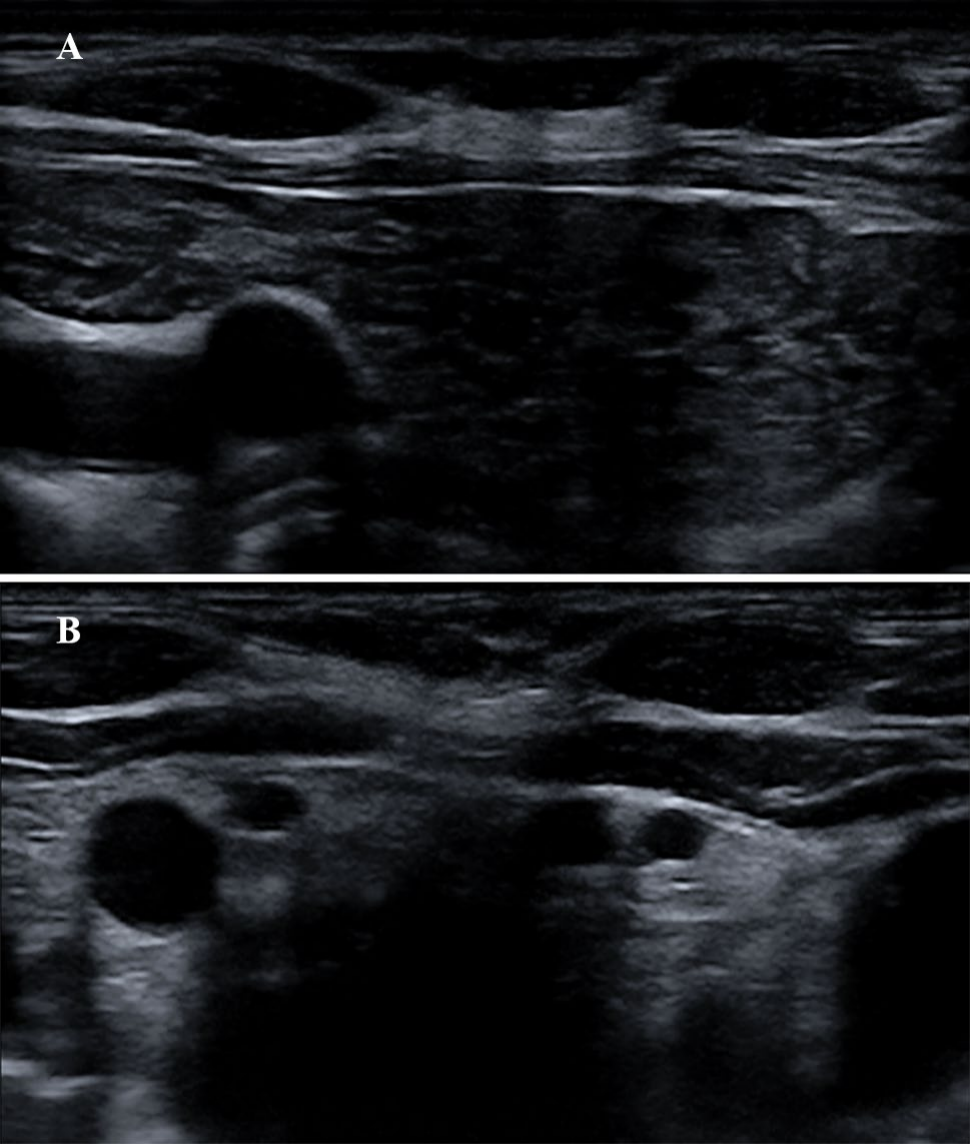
Representative case of GD-associated TH by nUS, at baseline (a) and after achieving stable euthyroidism (b). a 29-year-old woman with GD-associated TH: at diagnosis, thymus is sensibly enlarged in nUS transversal projection; b after 6 months of methimazole-based therapy under stable euthyroidism, the thymus parenchyma is not seen any more at nUS.*TH* thymic hyperplasia, *GD* Graves’ disease, *nUS* neck ultrasound
